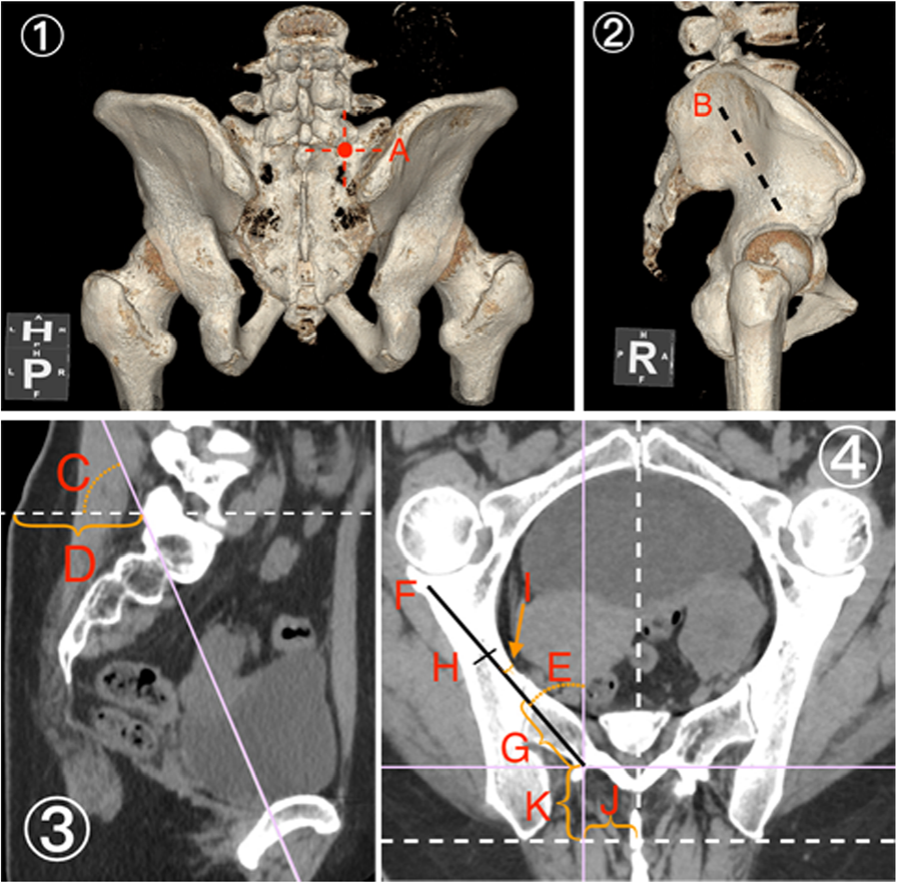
Case 7, 51-year-old female. 1 Posterior view of CT 3D imagine. A The entry point of S1AI trajectory. 2 Lateral view of CT 3D imagine. B The direction of S1AI trajectory. 3 Sagittal reconstructed plane of S1AI trajectory. C Sag. A.: 66.7°; D Skin D.: 63.8 mm. 4 Transverse plane of S1AI trajectory. E Tsv. A.: 40.3°; F Max. L.: 113.9 mm; G Sac. L.: 46.7 mm. H Iliac W.: 17.2 mm; I Cortex D.: 5.6 mm; J Mid. D.: 24.2 mm; K PSIS D.: 29.4 mm
